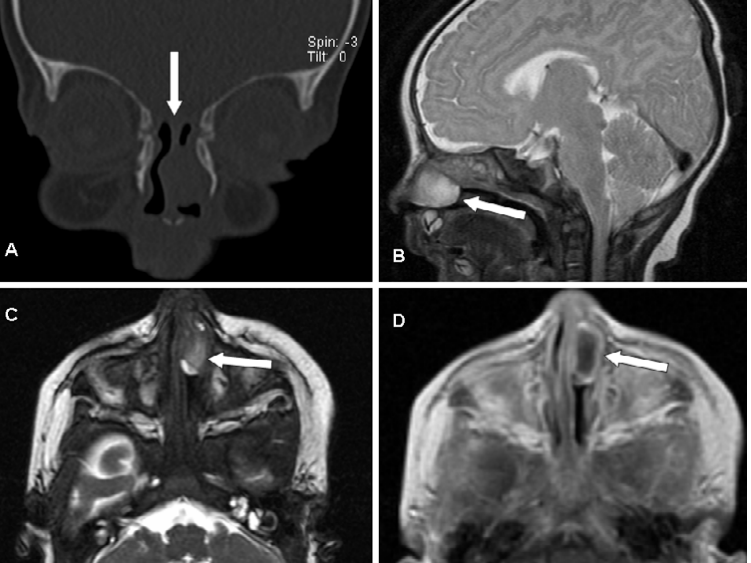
Nasal glial heterotopia. A: Coronal reconstruction of a spiral CT scan. A soft tissue mass is visible in left nasal cavity. Note the incomplete ossification of the skull base (arrow) at the age of 48 days. Ossification is regularly completed in this area at the age of 3 months. B: Sagittal T2 weighted MR image revealing an endonasal hyperintense mass without intracranial connection. C and D: axial T2 weighted and contrast-enhanced T1 weighted MR images showing a not contrast-enhancing mass in the nasal cavity. The rim-like contrast enhancement around the tumor is attributed to peritumoral mucosal swelling.
